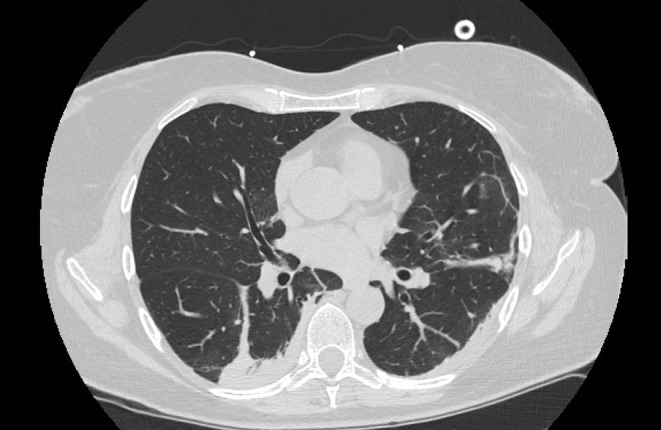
Typical CT findings in patients with COVID-19 pneumonia. Peripheral ground glass lesion with consolidations and bronchiectasis.
